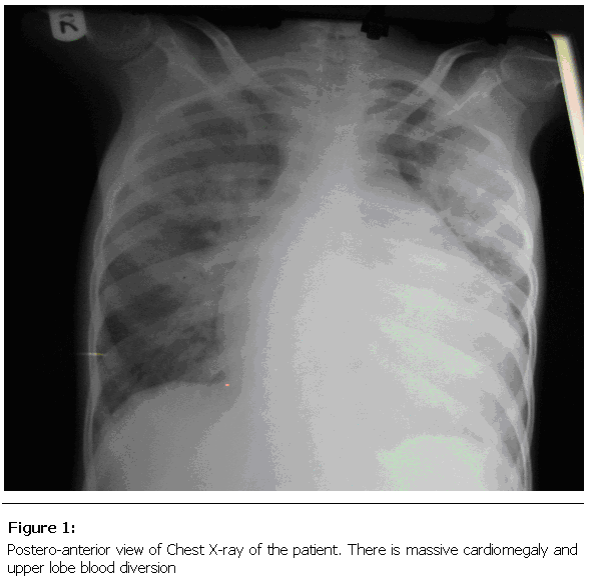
Postero-anterior view of Chest X-ray of the patient. There is massive cardiomegaly and upper lobe blood diversion.
